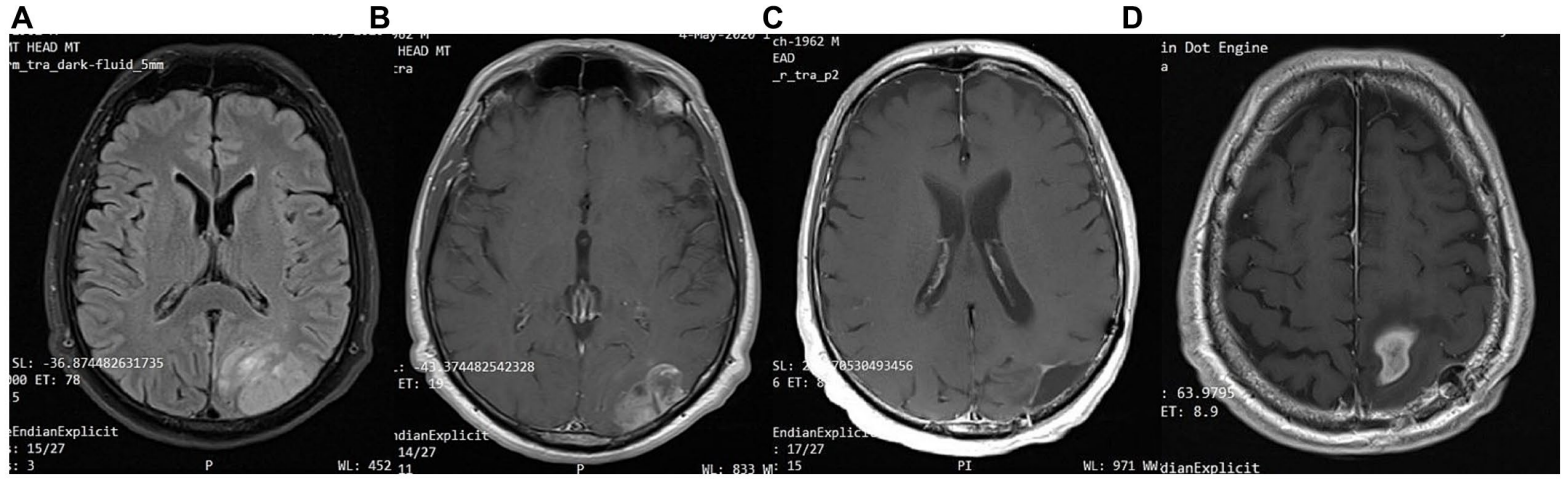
Patient 6: (A) brain MRI on diagnosis (T2/FLAIR) (B) brain MRI on diagnosis (T1 with contrast) (C) 18-month follow up brain MRI (T1 with contrast) (D) 24-month follow-up brain MRI (T1 with contrast).
